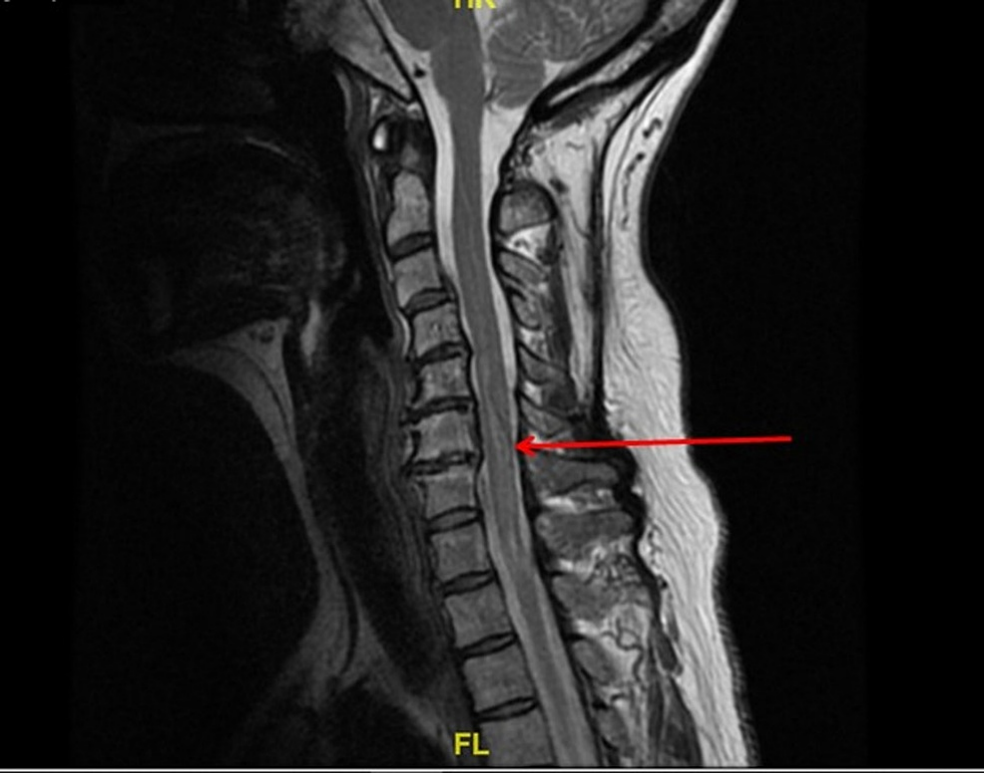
Sagittal, T2 MRI of the cervical spine. Red arrow indicates increased signal within the central gray matter across C6-T2.
